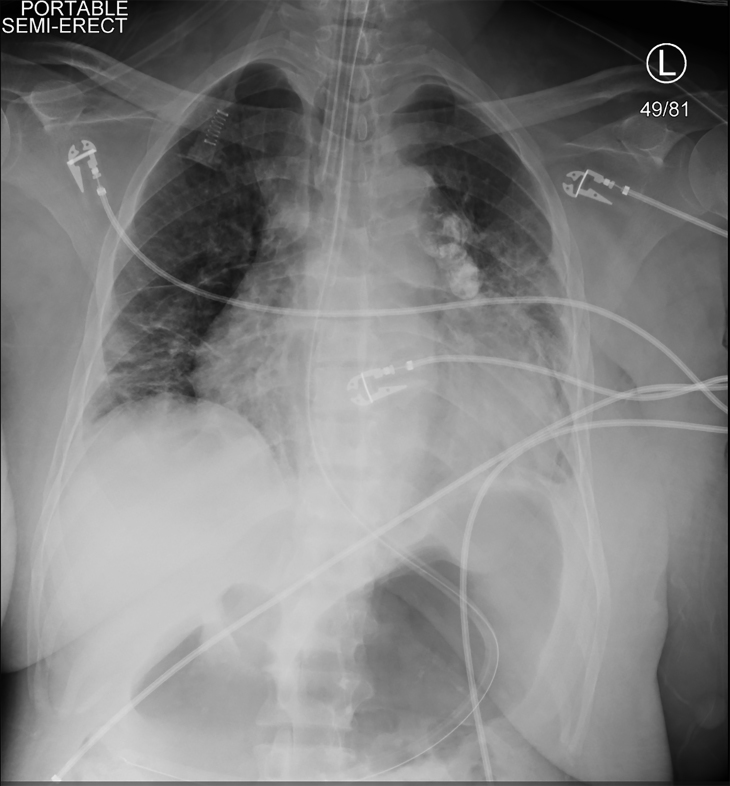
Post-intubation chest radiograph (posteroanterior view) showing proper endotracheal and nasogastric tube placement
